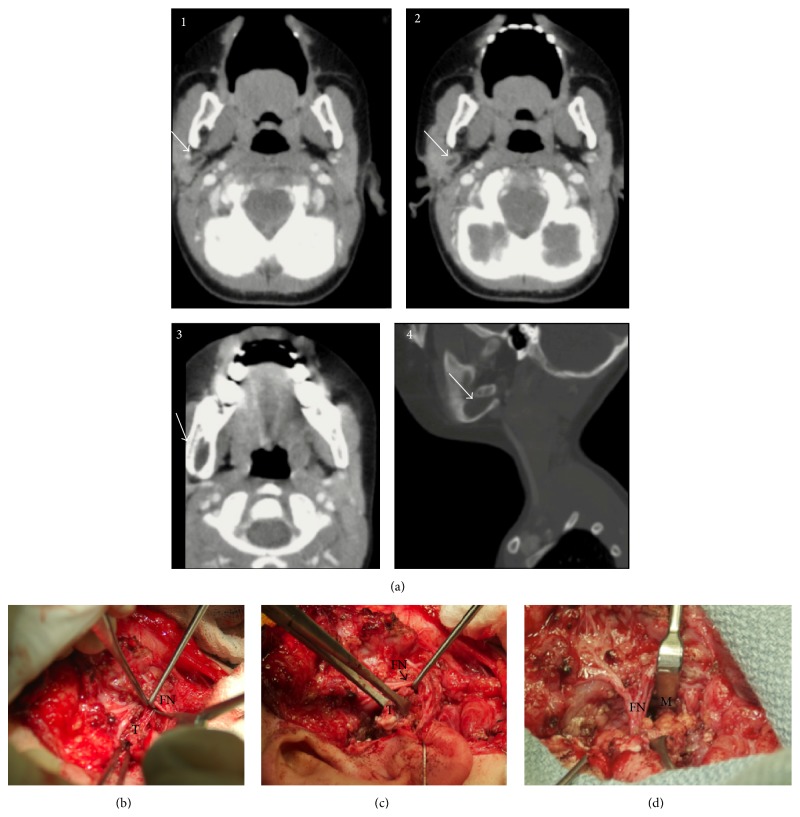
Case  3. (a)(1–4) Preoperative CT scans. White arrow depicts the right-sided lesion. (a)(1-2) Representative axial images revealing a well-defined right neck cyst (arrow) located posterior and deep to the parotid gland, with no clear connection to the skin. (a)(3-4) The extent of the right mandibular defect containing the cyst (arrow). (b) Identification of the tract extending deep to the facial nerve. In the inferior quadrant of the picture, a forcep is stenting the tract open as the facial nerve is being retracted. (FN: facial nerve; T: BCA tract). (c) Isolated tract after separating it from the facial nerve. A clamp is on the tract while the facial nerve is being retracted. (FN: facial nerve; T: BCA tract). (d) Tract extending medially entering the angle of the mandible. A clamp is on the tract (FN: facial nerve; M: mandible).
